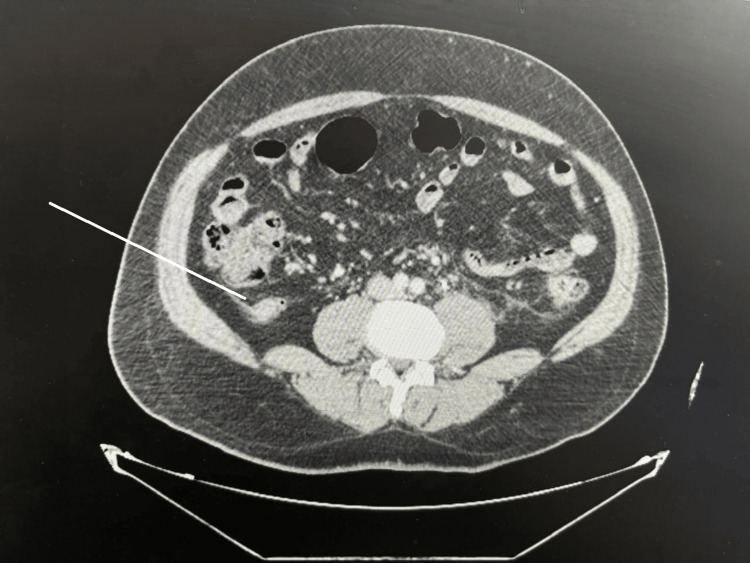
Abdomen CT scan image The image shows the 1.4 cm dilated appendix with fat stranding.
